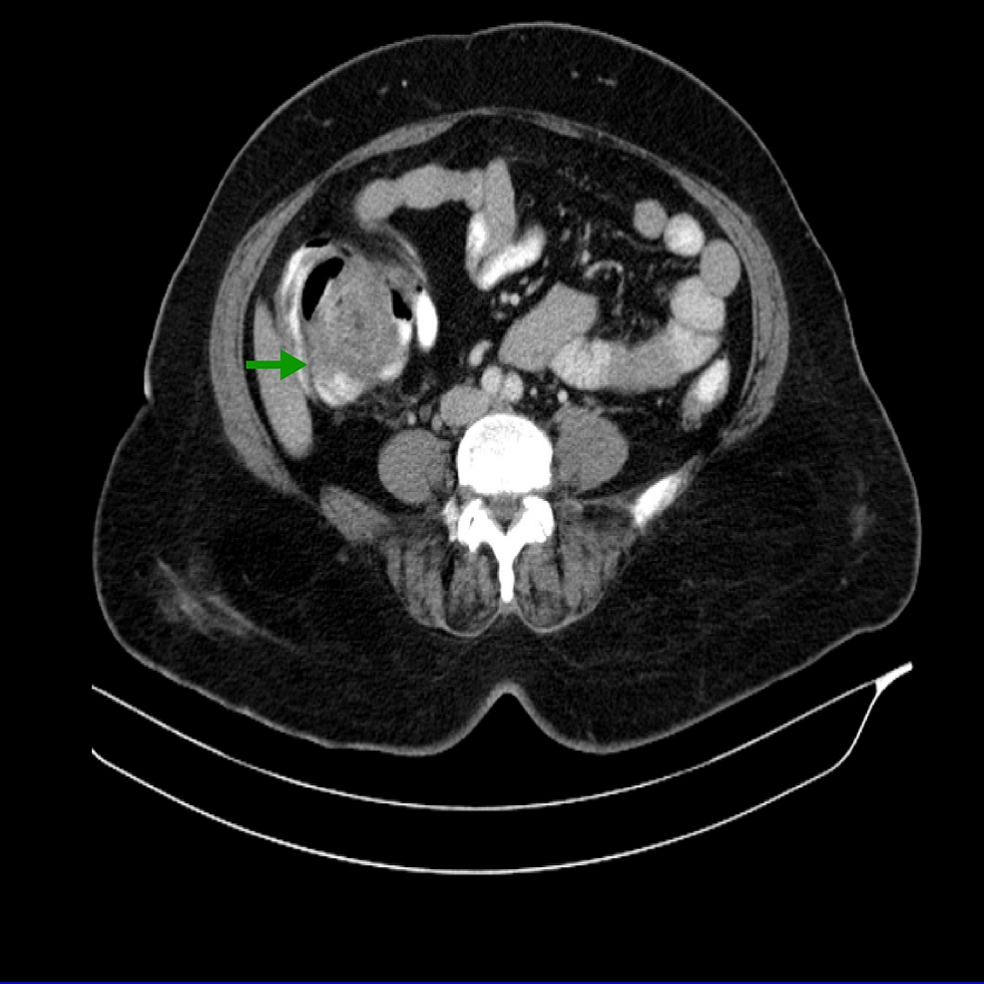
Abdominal CT scan image (axial section) The image shows a heterogenous soft tissue lesion causing intussusception, involving the cecum (green arrow).
